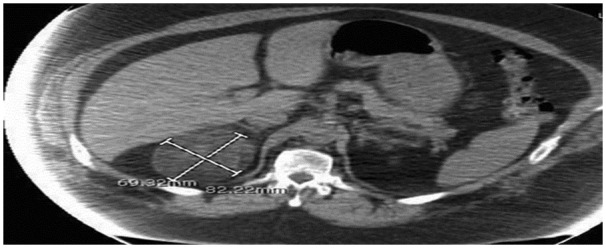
Computed tomography scan of the abdomen showing adrenal myelolipoma measuring
